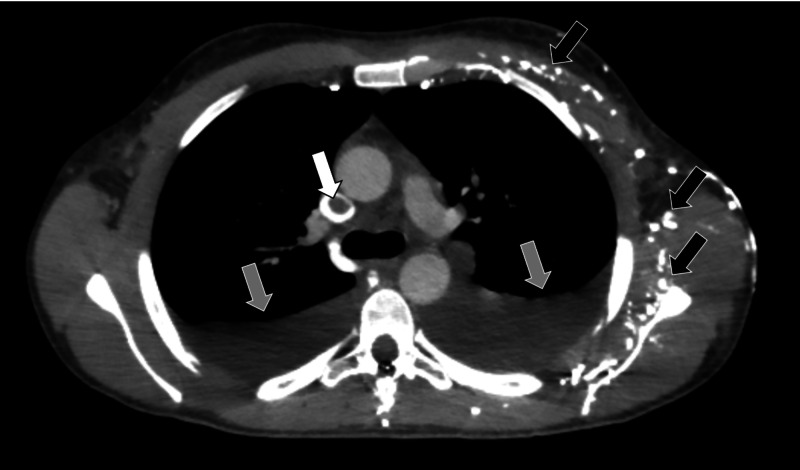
Contrast-enhanced CT of the chest Contrast-enhanced computed tomography of the chest showed suggestive signs of thrombus in the superior vena cava (white arrow), in addition to extensive venous ectasia in the left shoulder region (black arrows) and bilateral pleural effusion (gray arrows).
